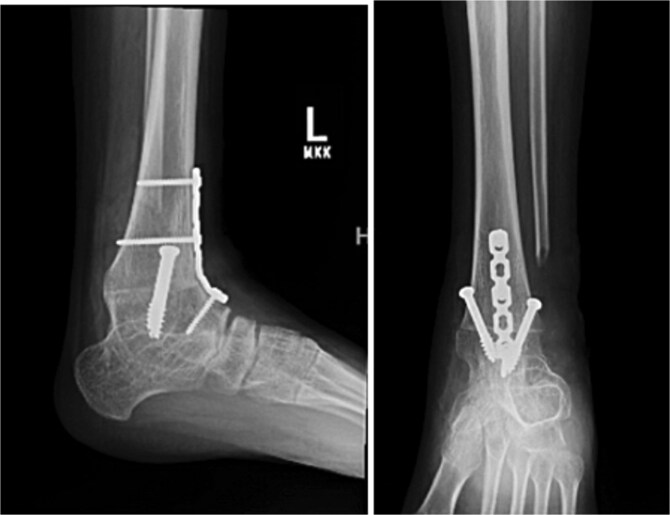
Postoperative radiographs of the left ankle and hindfoot following reconstructive surgery for rigid equinus deformity associated with subtalar coalition. The lateral and anteroposterior views demonstrate distal tibial and fibular osteotomies with internal fixation and improved hindfoot alignment.
